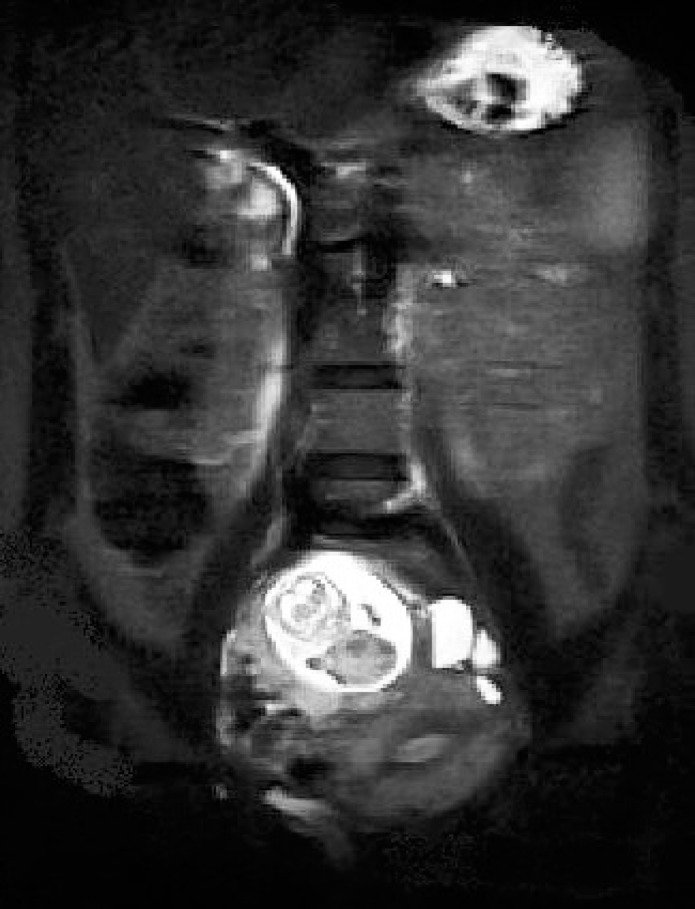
AbdominoPelvic MRI showing a large mass containing fetus in right adnexa in close contact with uterus.
